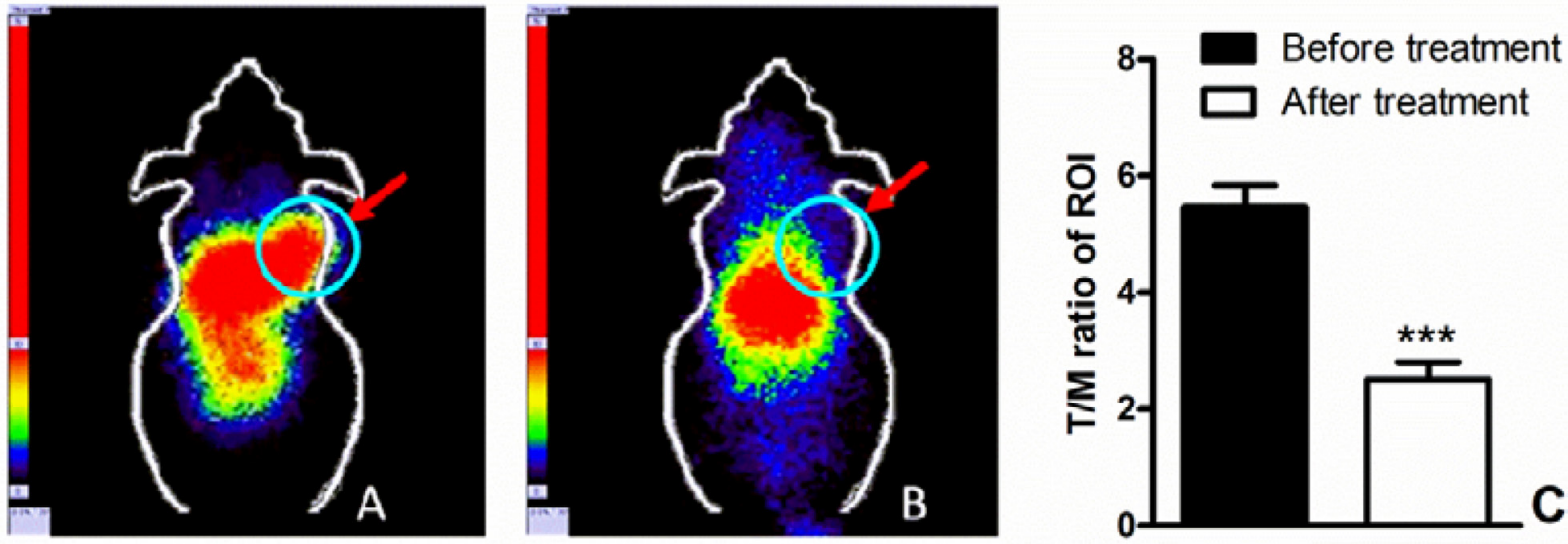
(A) 200 uci of 99mTc-HYNIC-MPG was injected via the tail vein, 2 h later, SPECT imaging was performed on PC9 xenografts; (B) 100 mg/Kg PD153035 was injected before SPECT imaging; T/M ratio before and after treatment was shown in (C).
